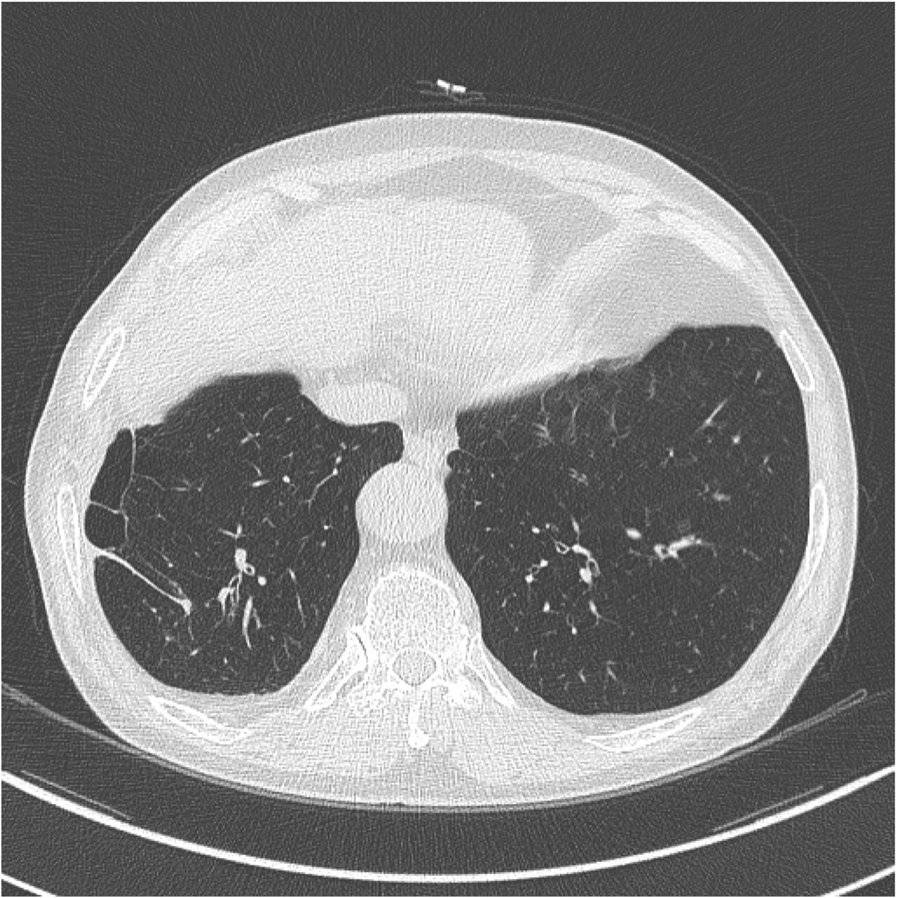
Computed tomogram image indicating peripheral bullous changes in the right lower lobe.
